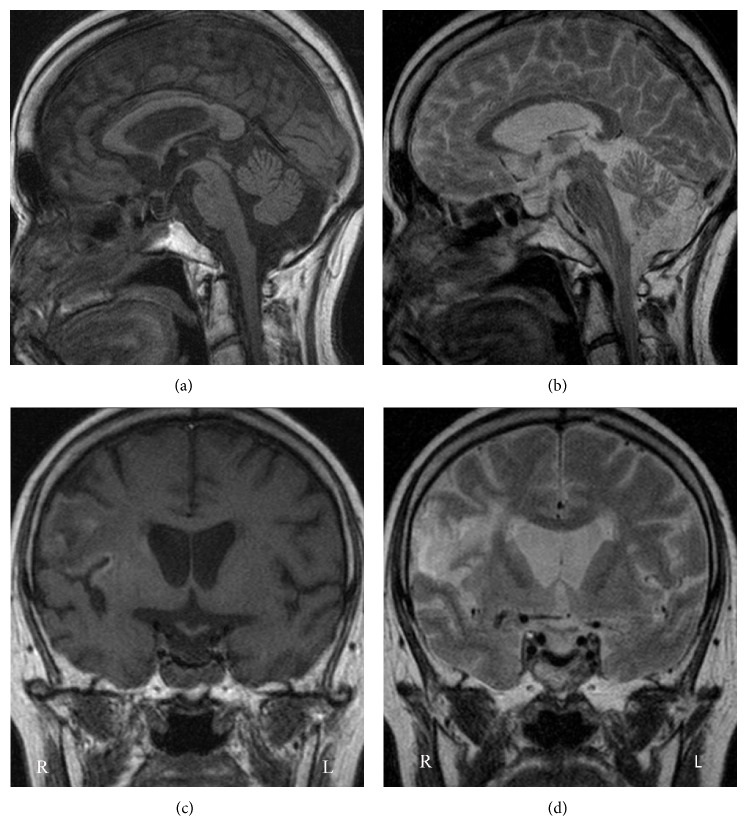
Sagittal and coronal views of the MRI showed the decompression of suprasellar region. (a, c) T1WI and (b, d) T2WI.
